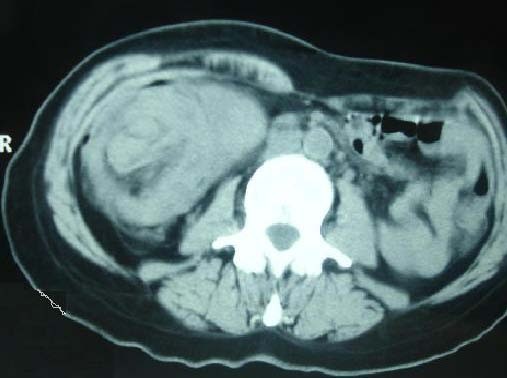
Axial CT of the abdomen shows the edematous wall of the intussuscipiens and the mass around the invaginating mesenteric fat.
